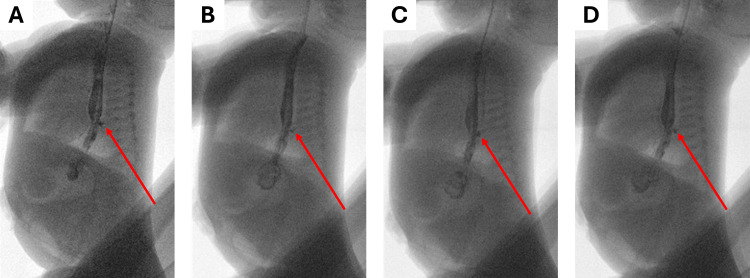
A radiocontrast evaluation performed two weeks after the final surgery showed a decreasing anastomotic leakage. (A) Initial X-ray taken upon an introduction of the contrast medium into the esophagus; (B) second X-ray taken after a waiting period; (C) third X-ray taken after a waiting period; (D) fourth X-ray taken after a waiting period. The time difference between each panel was at the discretion of the radiographer performing the evaluation. The red arrows point to the sites of radiocontrast leakage from the esophageal anastomosis.
